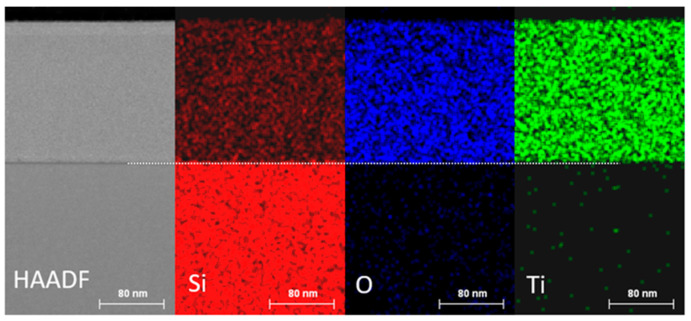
X-ray EDS mapping of the sample cross-section. The dark interface layer, is observed to be composed of only Si and O. This supports it is most likely the native oxide layer (SiO_x_) on Si substrate. The dotted line serves as a guide to an eye.
